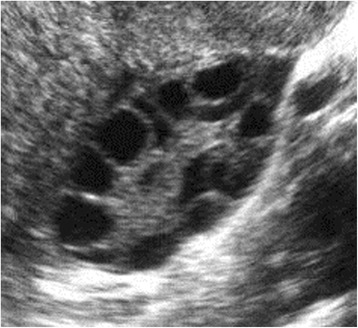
2D ultrasound image to determine antral follicular count. Legend: Ultrasound image by 2D conventional technique that allows the observer to count the number of antral follicles as well as determine its size and volume by *length × width × depth × 0.523*
